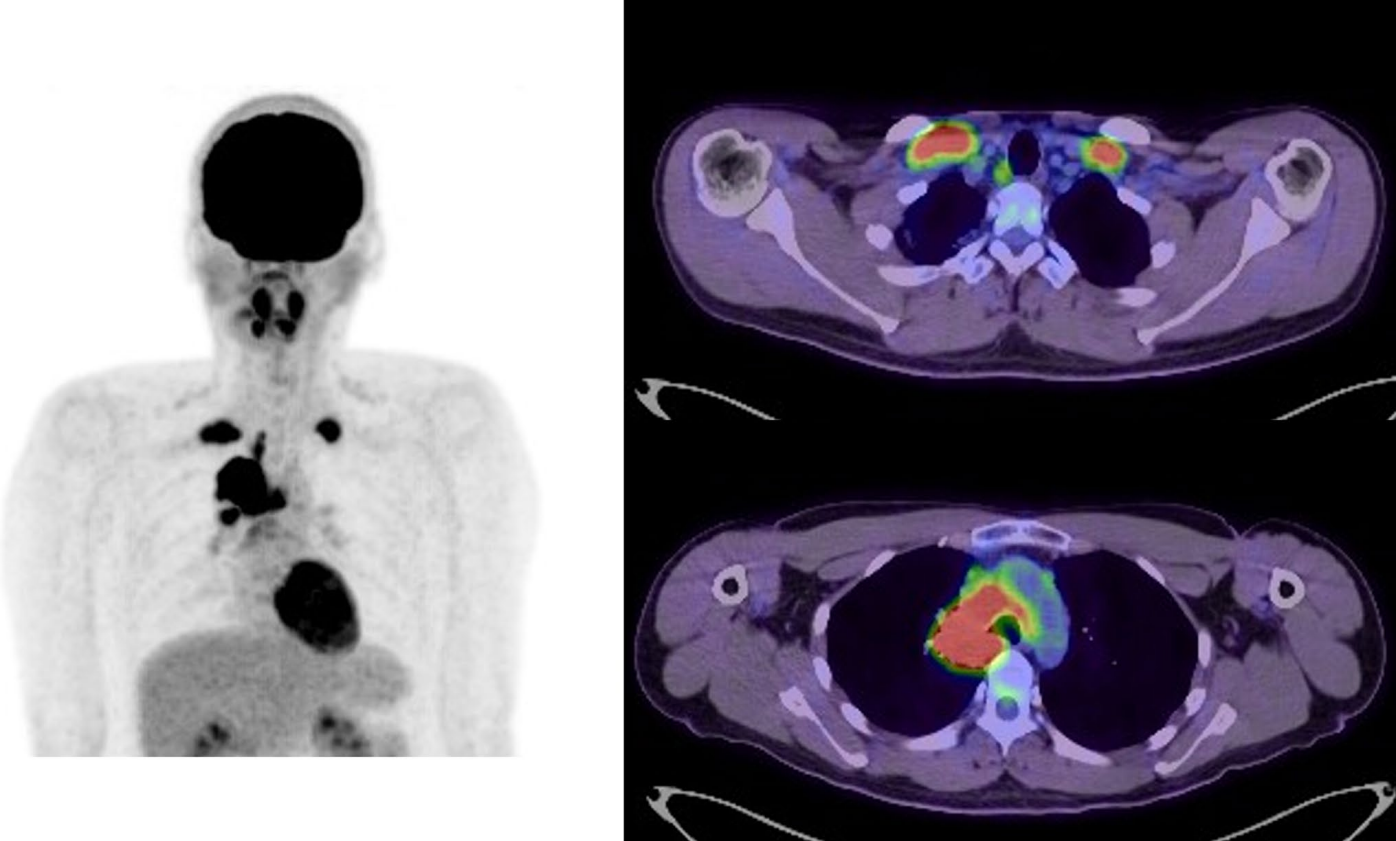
A case of right upper lobe lung cancer (cT3N3M0, stage IIIC). Fluorodeoxyglucose positron emission tomography/computed tomography revealed metastases in the right pulmonary hilum, right mediastinal lymph nodes, and bilateral subclavian lymph nodes
